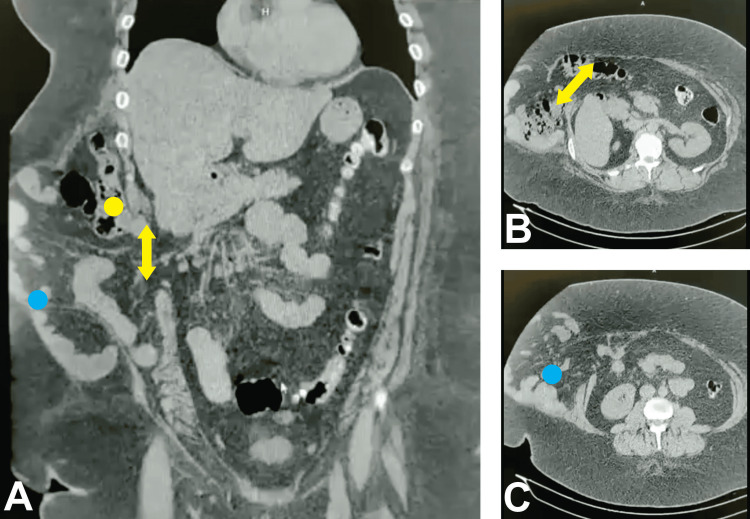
Preoperative Abdominal CT Imaging of the Incisional Hernia (A) Coronal plane demonstrating the hernial sac containing loops of the small intestine (blue dot) and colon (yellow dot) and the abdominal wall defect (yellow arrow, approximate width 12 cm). (B) Axial plane at the superior margin of the hernia defect (yellow arrow, approximate width 15 cm). (C) Axial plane at the inferior margin of the hernia defect (blue dot).
